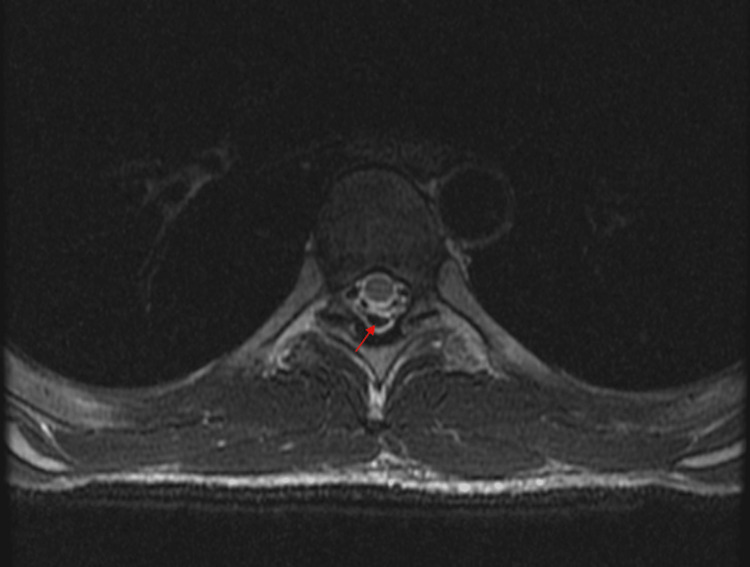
Axial magnetic resonance imaging of the spine demonstrating pneumorrhachis (red arrow)
